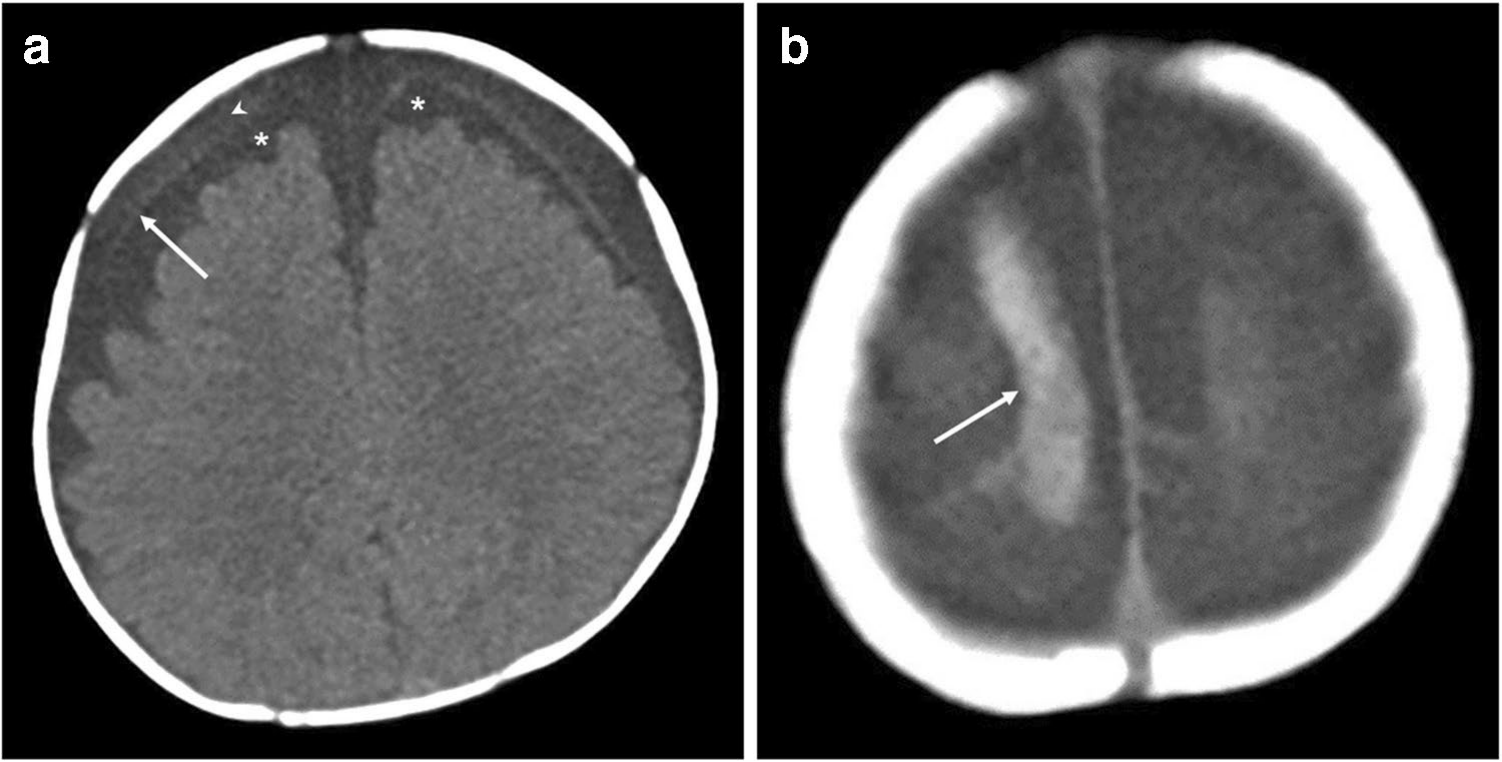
Selected axial head CT imaging (soft tissue window) in a 2-month-old boy with abusive head trauma demonstrates (a) bilateral benign enlargement of the subarachnoid spaces (*asterisks*) and bilateral subdural haemorrhages of mixed attenuation with slightly higher attenuation on the right (*arrowhead*). The arachnoid membrane separates the subdural collection from the enlarged subarachnoid spaces (*arrow*). b A rupture/thrombosis of a bridging vein at the right vertex (*arrow*)
